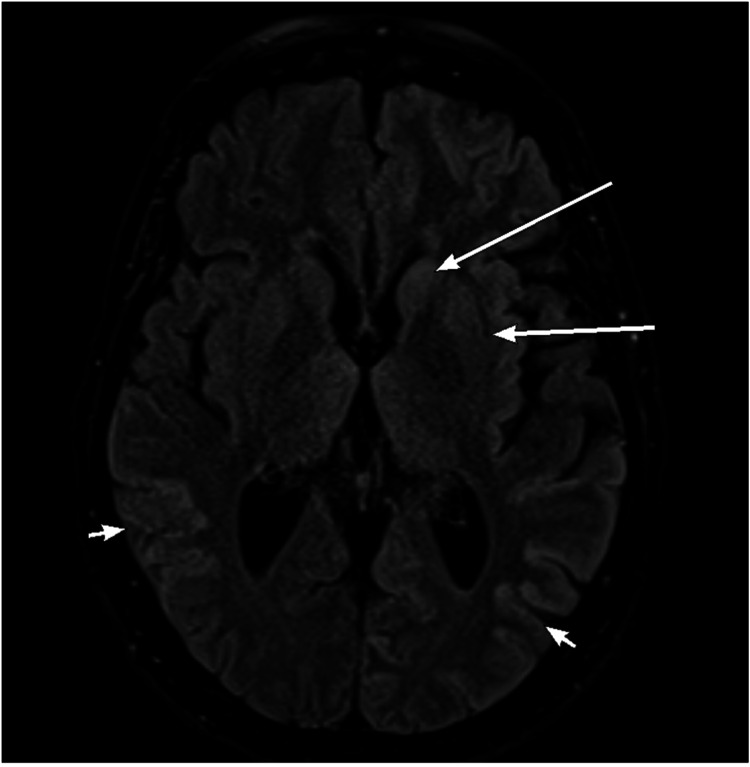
Fluid-attenuated inversion recovery (FLAIR) image of the brain showing subtle hyperintensity involving the parietal cortex bilaterally with an asymmetric pattern (small arrows), also involving the left caudate head and left putamen (long arrows).
